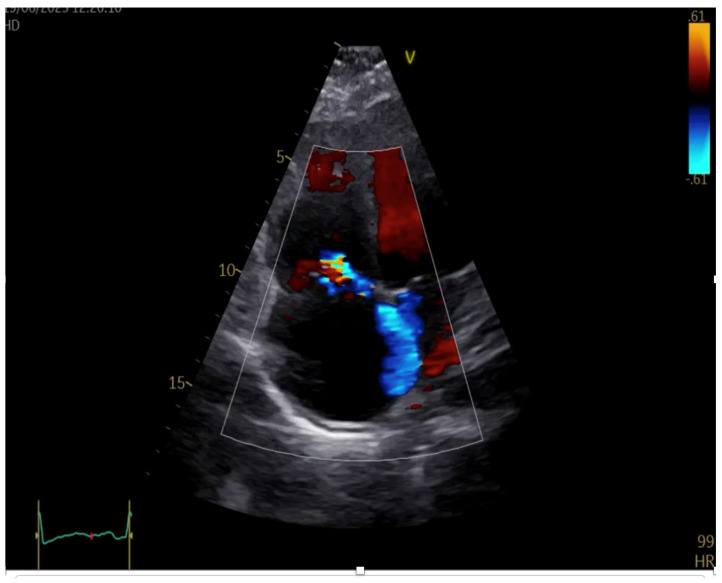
Transthoracic echocardiography at admission, apical four chamber view, showing severe tricuspid regurgitation.
